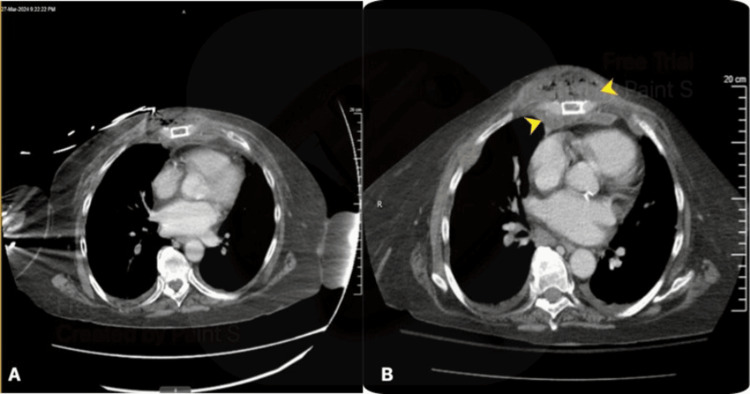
Computed tomography of the chest obtained on admission and preoperatively. Two axial images demonstrate the initial scan with a retrosternal hematoma (A) and subsequent preoperative abscess formation with extension into the mediastinum (B). Yellow arrows indicate the abscess formation with extension into the mediastinum.
